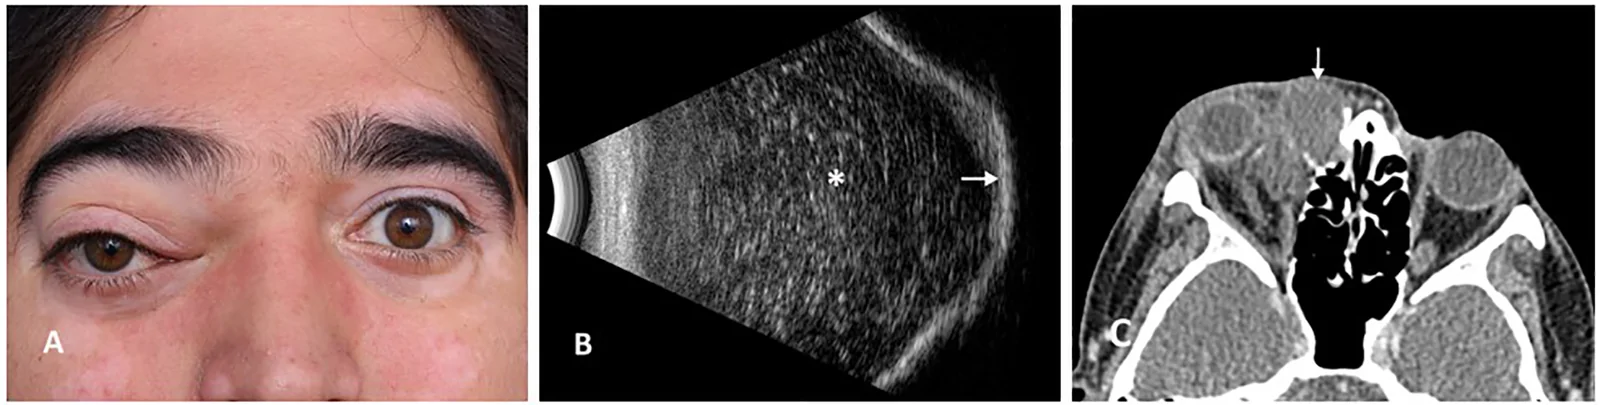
Orbital Mucocele. (A) External photography shows right upper lid swelling, proptosis, and inferior eye displacement in a patient presenting an orbital mass for one year. (B) Paraocular B-scan shows a well-circumscribed low reflective lesion (asterisk) with associated bone remodeling (arrow). (C) Axial CT scan shows a large expansile right fronto-orbital mucocele (arrow) and bone erosion. The asterisk (*) is used to label an area of the figure.
